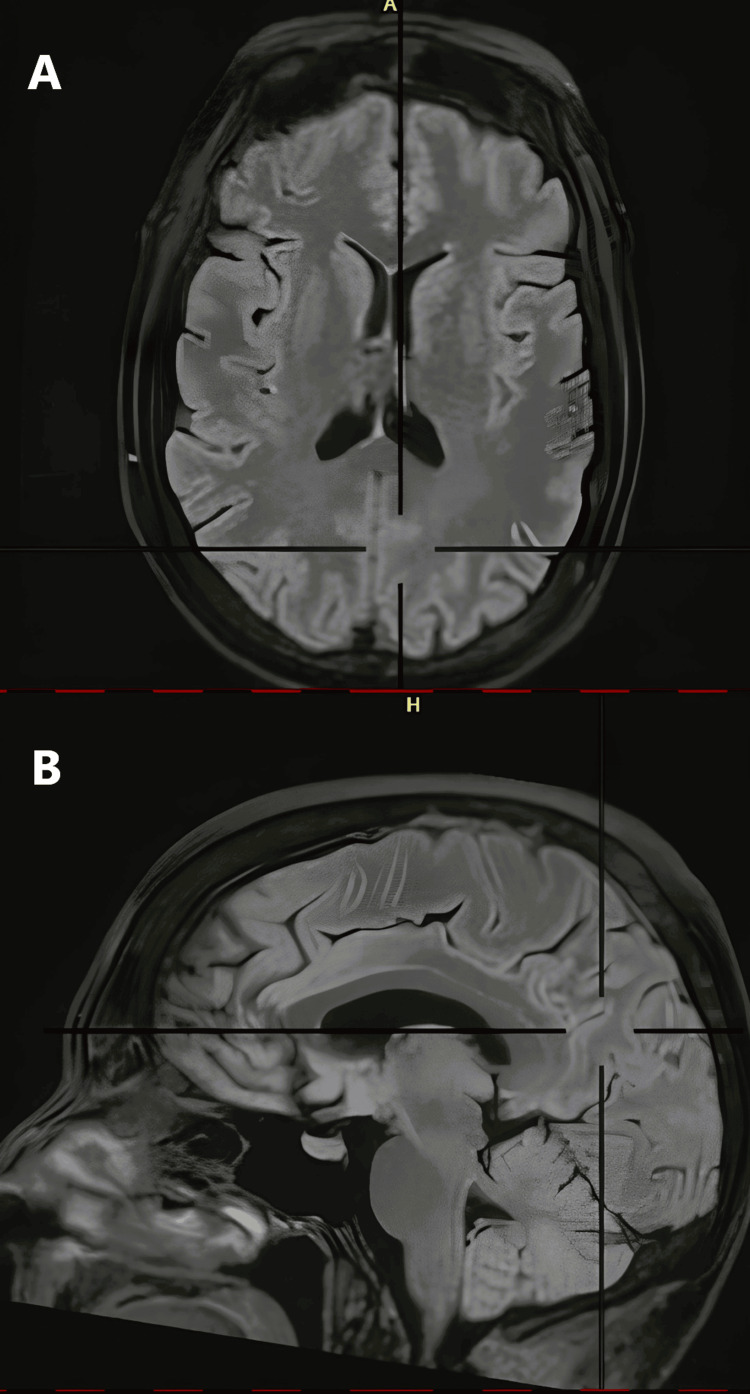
T2 FLAIR (A) axial and (B) sagittal views Resolution of multifocal high-signal intensity areas FLAIR: fluid-attenuated inversion recovery
